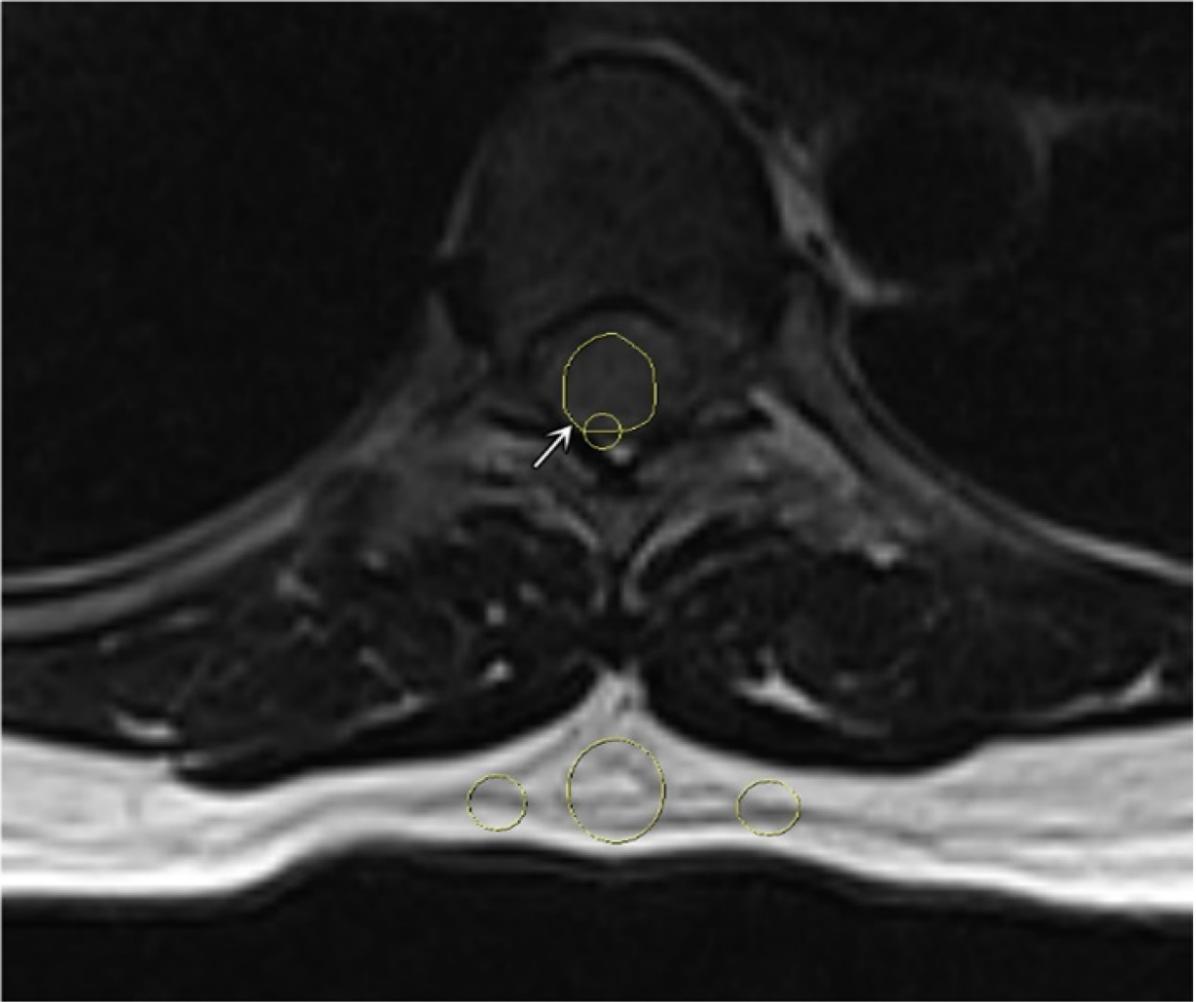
A representative region of interest on the tumor (white arrow) and 3 subcutaneous regions on axial T2-weighted image.
